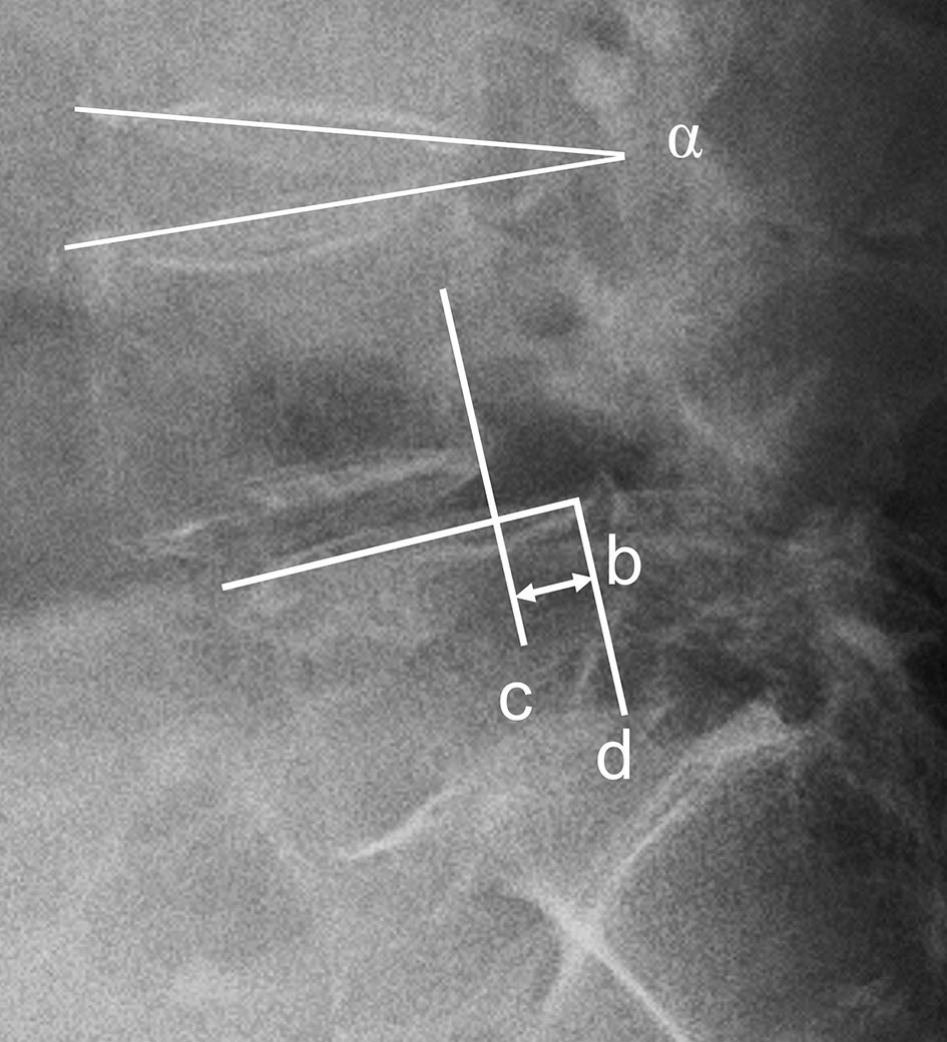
Radiographs of lumbar spine with spondylolisthesis to demonstrate the measurement technique. Sagittal translation (ST): first, the perpendicular distance between parallel lines c and d was measured on radiographs, the distance between these two parallel lines was the distance of slippage (b). The difference of the distance of slippage between flexion and extension was ST. Sagittal angulation (SA): the intervertebral slip angle(α) was the angle between two lines at the endplates of the affected disc. The difference of intervertebral slip angles between flexion and extension radiographs was SA.
